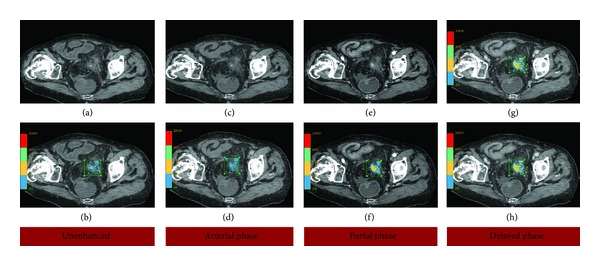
Oblique CT images before ((a), (b)) and after contrast medium administration in the arterial phase ((c), (d)), portal phase ((e), (f)), and delayed phase ((g), (h)) showing the foreign body and perifocal contrast-enhancement. In the lower images ((b), (d), (f), and (h)) a density color map has been superimposed using Advantage Workstation 4.5 (GE) to better depict the increase of HU in the different phases.
